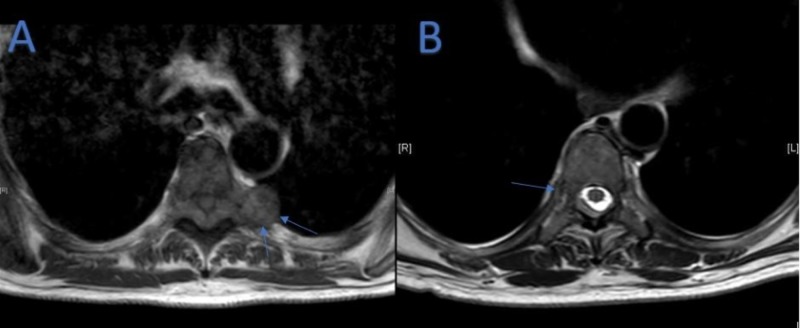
(A) Axial Magnetic Resonance Imaging (MRI) at T5 Vertebral Level on Day One of Admission. (B) Axial MRI at T8 Vertebral Level on Day One of Admission. (A) Gadolinium-enhanced T1-weighted axial MRI on day one of admission showing posterior epidural lesion at T5 vertebral level (arrows). (B) Gadolinium-enhanced T2-weighted axial MRI on day one of admission showing small lesion at T8 vertebral level involving right posterior aspect of T8 vertebral body (arrow).
